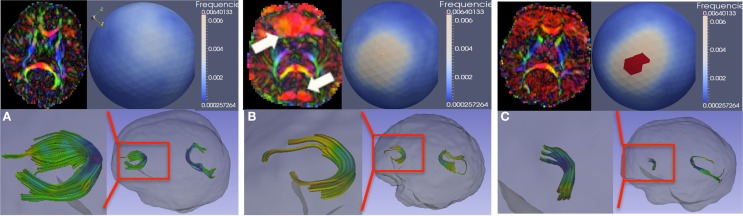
Vibration artifacts. (A) Artifact free scan. Top-left, a representative axial slice of the color-FA map. Top-right, spherical histogram of the PD distribution within the entire brain. Bottom-right, tractography of genu and splenium of the corpus callosum. Bottom-left, genu tract in more detail. (B) Vibration artifacts may manifest as localized (prefrontal region for this example) signal-loss in the DWI image or as dominant L-R (red) direction. (C) Vibration artifact in the absence of localize DWI signal loss. Spherical viewpoints chosen to show locations of highest histogram frequency.
